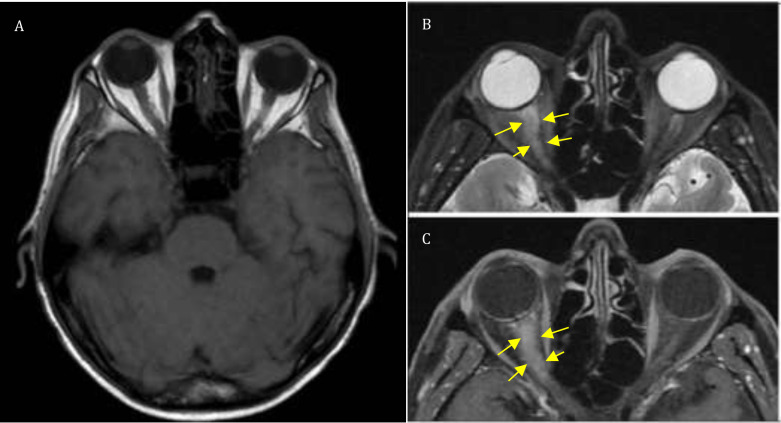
Two different MRI brain images comparing optic nerve enlargement. (A) Patient’s initial brain MRI without optic nerve thickening. (B, C) Images showing right optic nerve enlargement (referenced from the Indian Journal of Ophthalmology—Case Reports 3(3):873–876, Jul–Sep 2023). *Yellow arrows* indicate the areas of enlargement.
